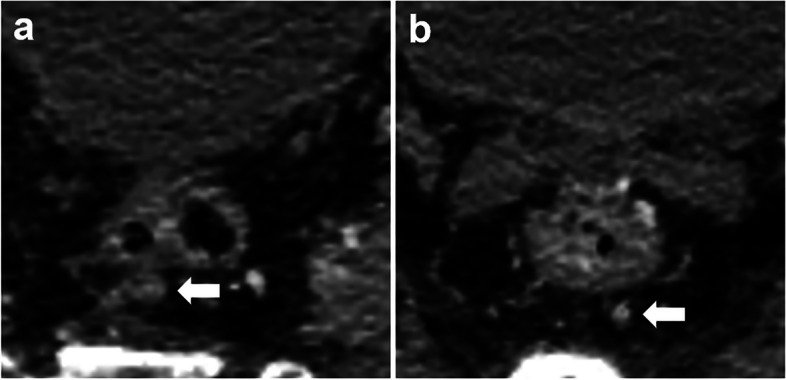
portal venous phase CT images of a 68-year-old male with moderately differentiated pathologic T3N + rectal adenocarcinoma. a The size-prioritized lymph node (indicated by a white arrow) exhibits a short-axis diameter of 5 mm, with ill-defined borders, heterogeneous enhancement, and an elliptical shape. It has been assigned a Node-RADS score of 4. b The morphology-prioritized lymph node (indicated by a white arrow) shows a short-axis diameter of 4 mm, with unclear borders, heterogeneous enhancement, and an irregular shape. It has been assigned a Node-RADS score of 3. The Node-RADSmax score for this patient is 4
